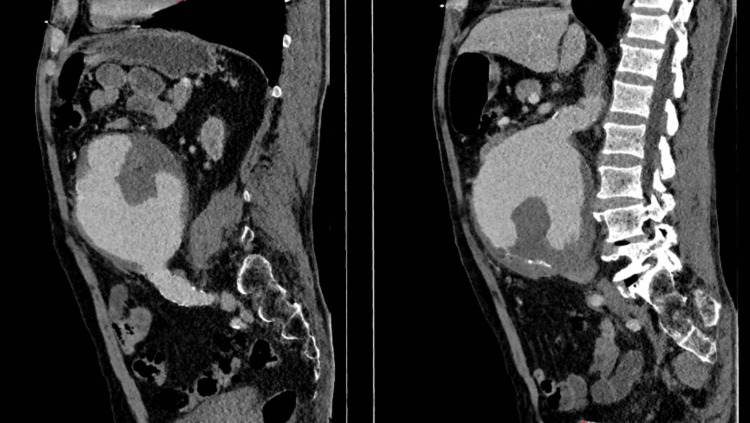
Contrast-enhanced CT (sagittal reconstruction) demonstrating a large infrarenal AAA Contrast-enhanced CT (sagittal reconstruction) showing a large infrarenal abdominal aortic aneurysm with eccentric mural thrombus and a centrally opacified residual lumen, without evidence of active contrast extravasation. AAA, abdominal aortic aneurysm
